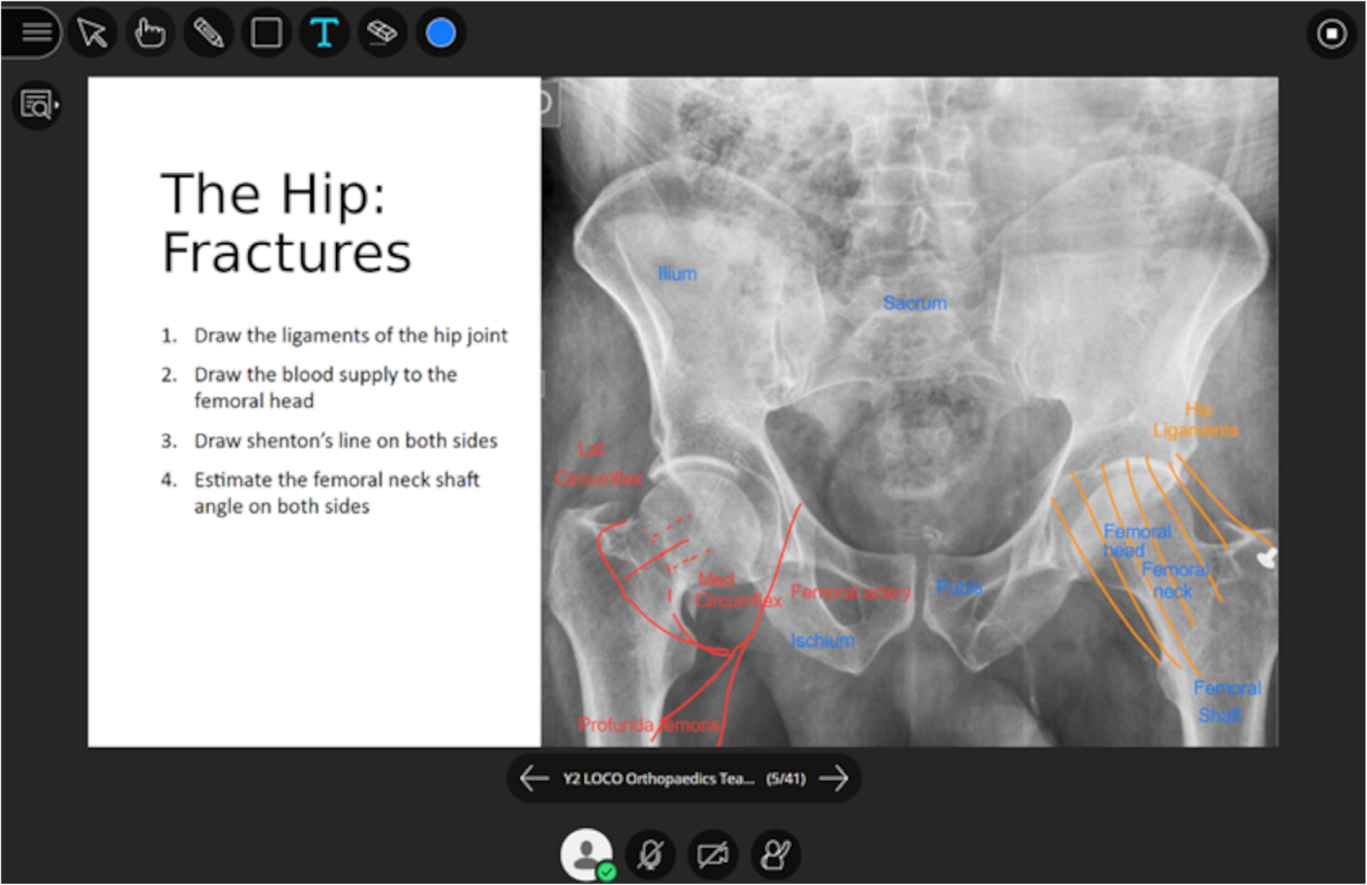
An example of collaboration in BBC. Students work together to label and interpret the positions of anatomical structures on a radiograph. BBC, Blackboard Collaborate [Color figure can be viewed at wileyonlinelibrary.com]
